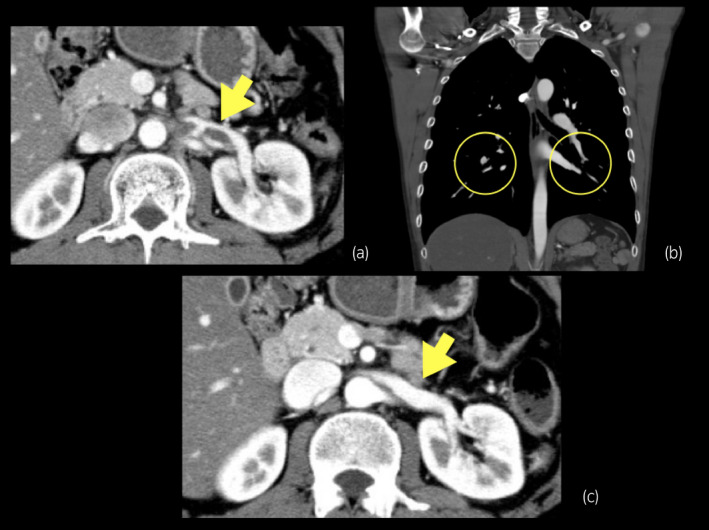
Ten days after the initiation of rivaroxaban, CTA revealed a reduction in the size of the left RVT (a) and disappearance of the PTE (b). Twenty days after initiation of rivaroxaban, CTA revealed complete disappearance of the left RVT (c).
